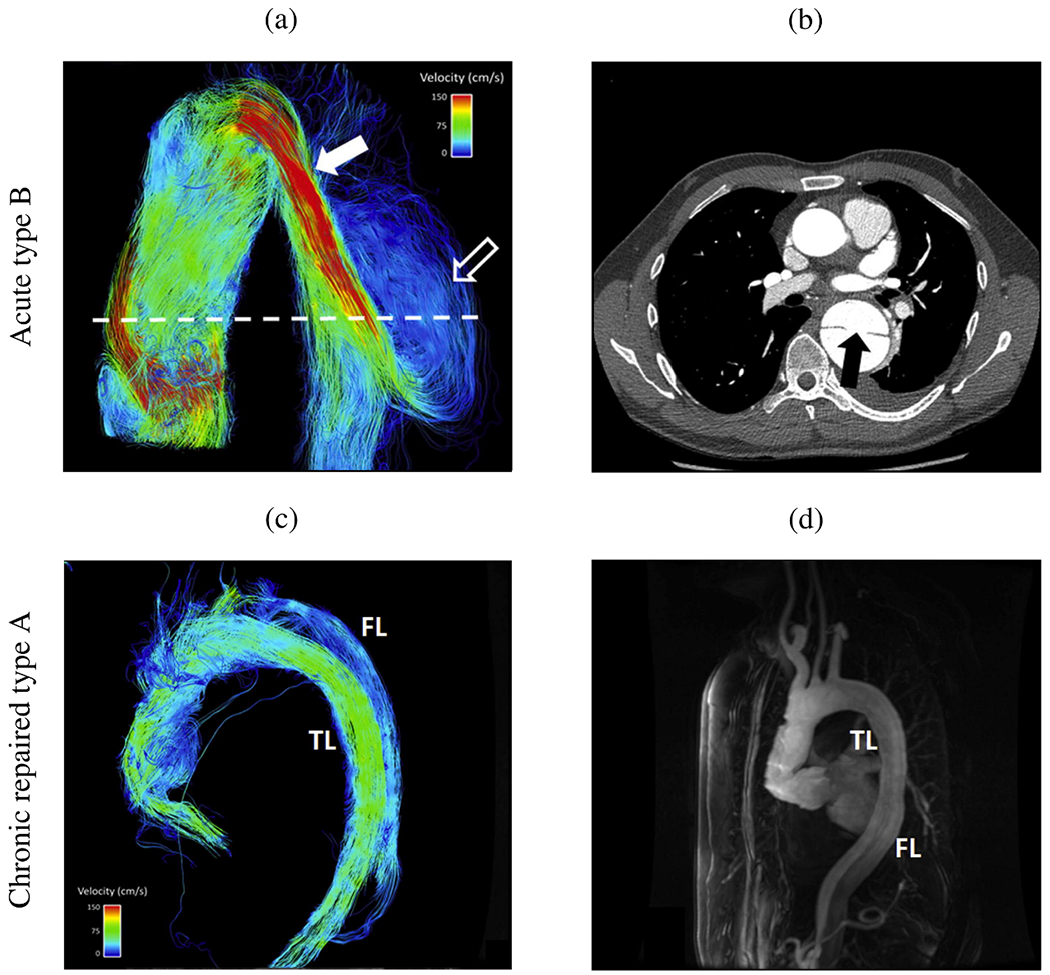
A representative case of acute type B dissection shows (a) a color-coded streamline visualization from 4D-flow MRI during mid-systole, highlighting flow acceleration (open arrow) in the proximal descending aorta directed into the false lumen through a large intimal tear ((b), black arrow), seen on axial CTA at the indicated level (dashed line), with false lumen flow characterized by a large vortex ((a), white arrow), resulting in predominantly retrograde flow. A representative case of chronic repaired type A dissection shows (c) a color-coded streamline visualization during mid-systole revealing slow flow through the false lumen (‘FL’) compared to the true lumen (‘TL’). This can also be seen in the subtracted maximum intensity projection images (d) acquired with time-resolved contrast-enhanced MRA. Figure is adapted from Francois et al. [159] with permission from Elsevier.
